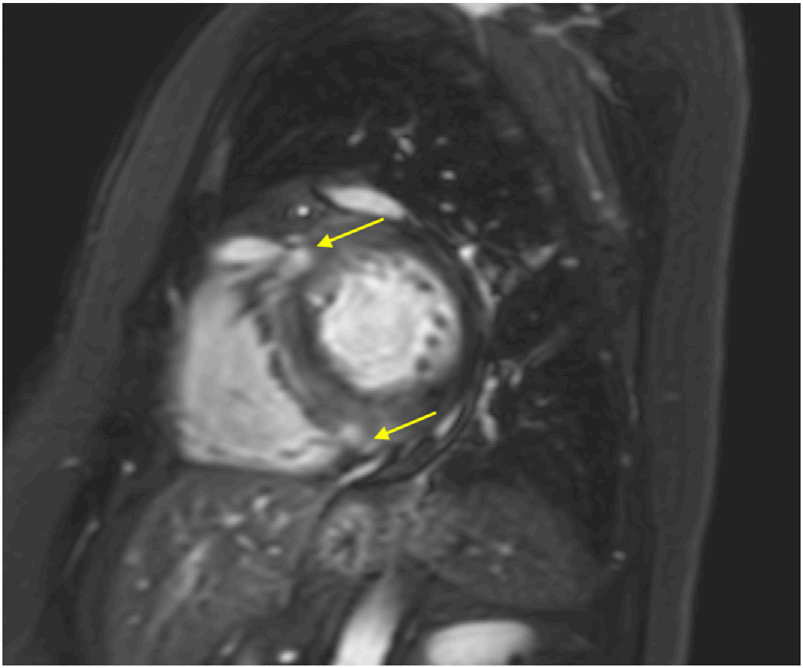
Cardiac MRI of case 2. Cardiac magnetic MRI showing LGE in the septum at the right ventricular junction (yellow arrows).
